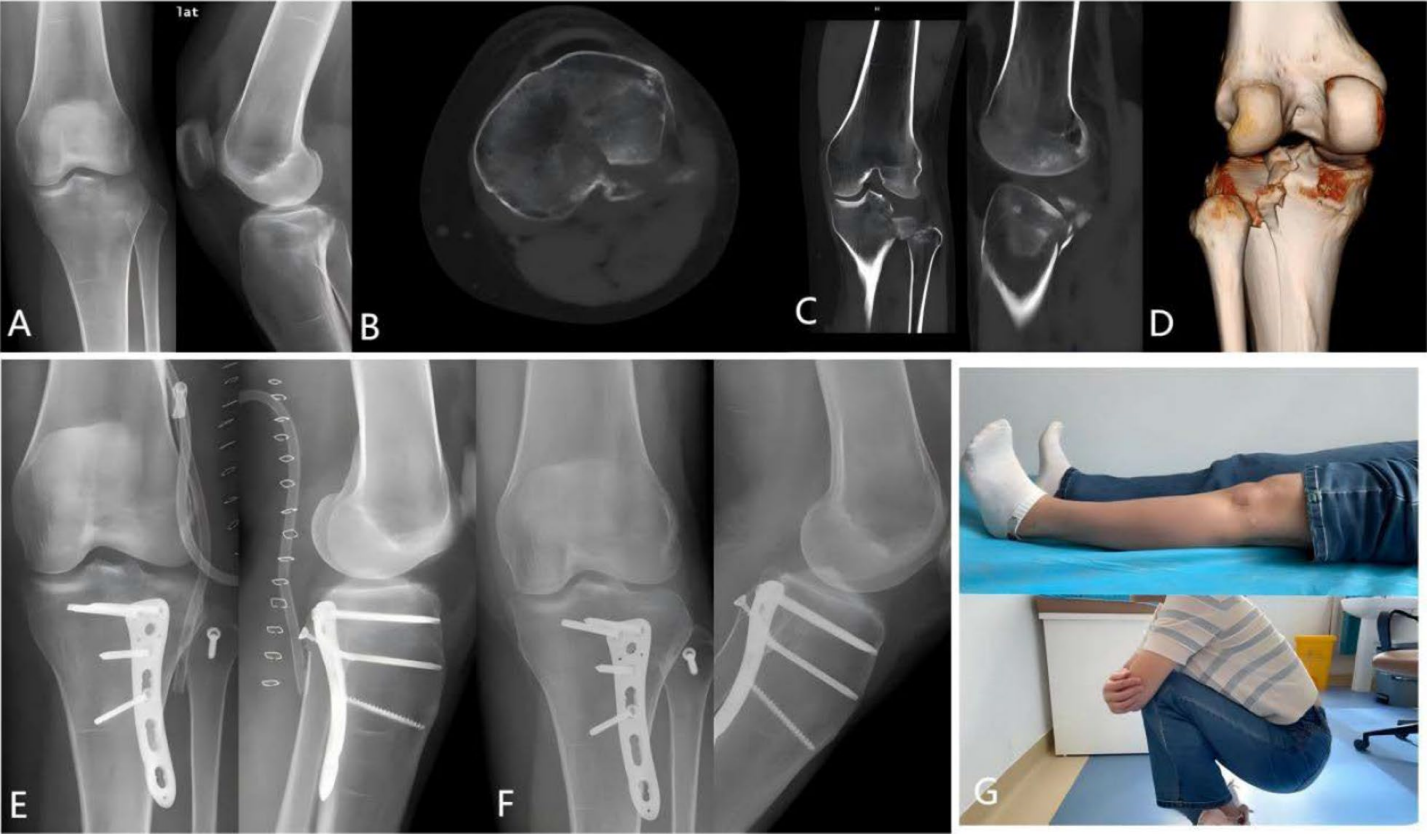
A case of a posterolateral tibial plateau fracture classified as Type III PL according to the Kfuri–Schatzker classification. A Preoperative anteroposterior and lateral X-rays of the affected knee joint. B–D Preoperative CT scans with 3D reconstruction showing significant depression of the posterolateral articular surface and rupture of the posterior wall. E Postoperative anteroposterior and lateral X-rays of the knee joint showing perfect fracture reduction. F Anteroposterior and lateral X-rays at 6 months postoperatively showing healed fracture and osteotomy sites. G Clinical photograph at 1 year postoperatively demonstrating good functional recovery of the knee joint
